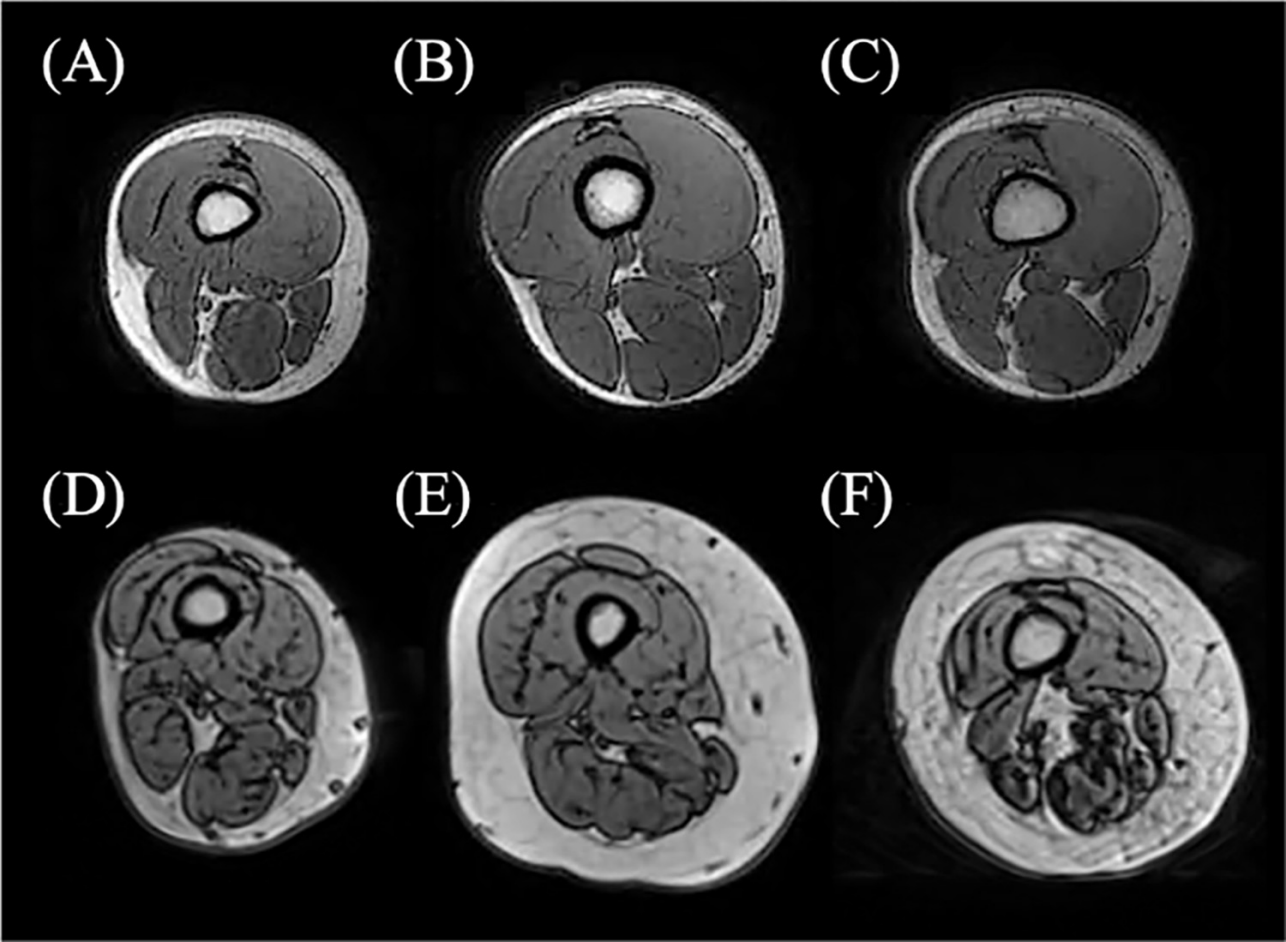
Comparison of thigh MRI for young healthy individuals (A, B, C) and older individuals (D, E, F).
